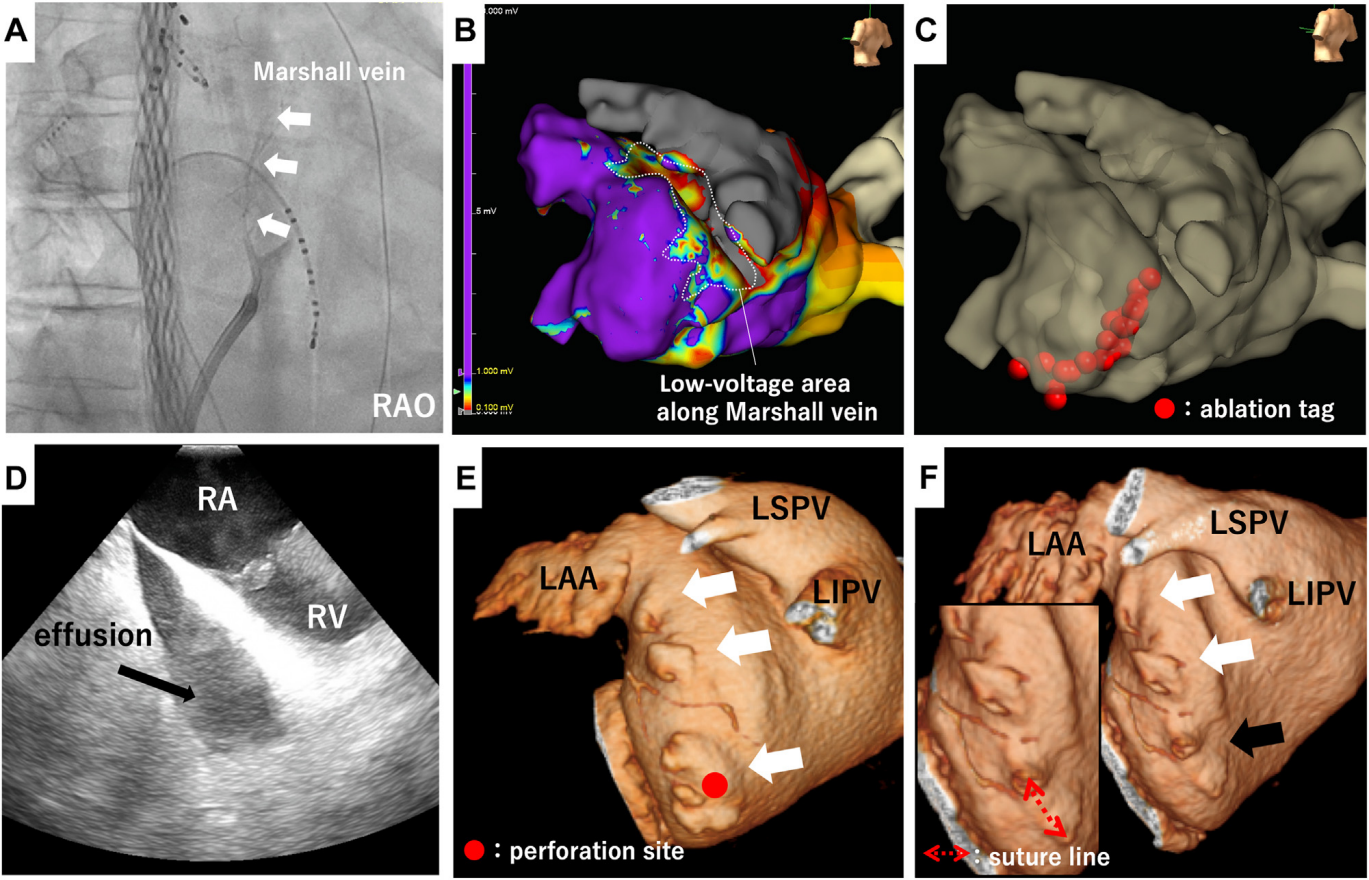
(A) Imaging of the Marshall vein before ethanol injection (white arrows) in an RAO view. (B) Voltage map after ethanol injection into the Marshall vein. A low-voltage area is observed along the ridge between the LAA and left pulmonary veins. (C) Ablation line on the mitral isthmus. (D) Pericardial effusion observed on intracardiac echocardiography. (E) 3D-CT image around the mitral isthmus before ablation, showing multiple accessory LAAs (white arrows). (F) 3D-CT image around the mitral isthmus after ablation. The largest LAA appears to have been sutured during the cardiac surgery (black arrow). 3D-CT, 3-dimensional computed tomography; LAA, left atrial appendage; LIPV, left inferior pulmonary vein; LSPV, left superior pulmonary vein; RA, right atrium; RAO, right anterior oblique; RV, right ventricle.
